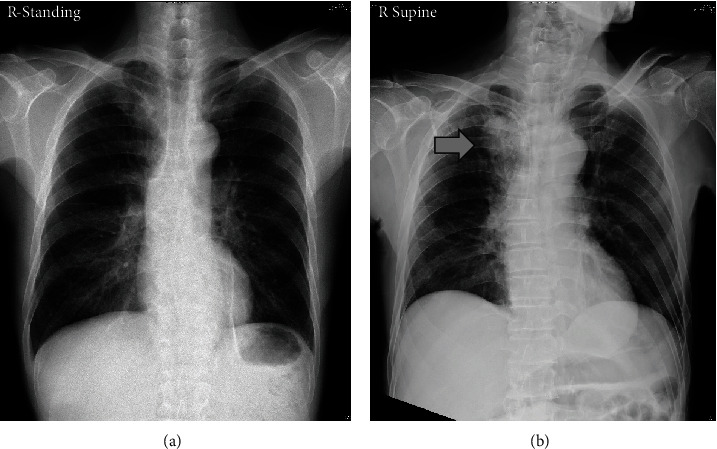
Unremarkable chest X-ray at (a) mania onset and (b) 2 years later, with a mass (arrow) in the right upper lobe of the lung.
